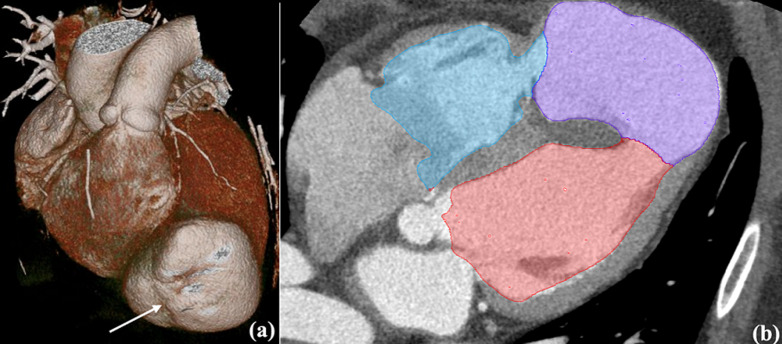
cardiac computed tomography angiography (CCTA); A) 3D reconstruction, where pseudoaneurism is shown with an arrow, and B) four-chamber long-axis view - showing apical pseudoaneurysm (violet) connected to the left ventricle cavity (red) and to the right ventricle (blue); dilated chambers and serous pericardial effusion are also seen
